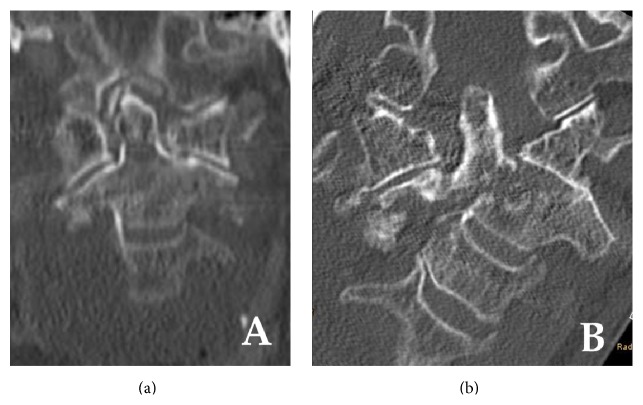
Coronal plane cervical spine computed tomography (CT) images of a 90-year-old woman. (a) Initial imaging showing minimal displacement and good cancellous bony contact and the typical oblique fracture pattern. (b) Follow-up imaging after 6 weeks of collar immobilization showing severe lateral displacement and tilting with dislocation of the left C1/2 facet joint.
